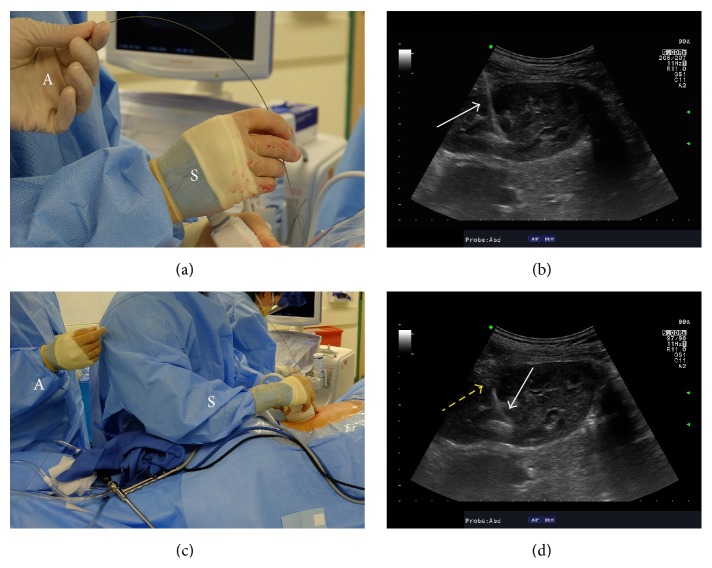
Introduction of working wire and dilators using ultrasound guidance. (a) A J-tip coaxial wire is inserted through the needle after its tip is confirmed in the collecting system. The operative surgeon (S) controls the needle with dominant hand and holds the ultrasound probe with the nondominant hand while the assistant (A) controls the wire. (b) Ultrasonographic image of the kidney demonstrating the wire (white arrow) in the collecting system after needle withdrawal. Its appearance is highly echogenic and prominent. (c) For fascial dilation, a 10-French fascial dilator is passed over the wire. Again, the operative surgeon (S) holds the ultrasound probe and controls the dilator while the assistant (A) controls the wire. (d) As the fascial dilator passes over the working wire, the wire is seen very clearly as an echogenic line (white arrow). The dilator obscures the view of the wire and this interface of the echogenic wire and the area where the wire disappears from view is the tip of the fascial dilator (yellow arrow).
